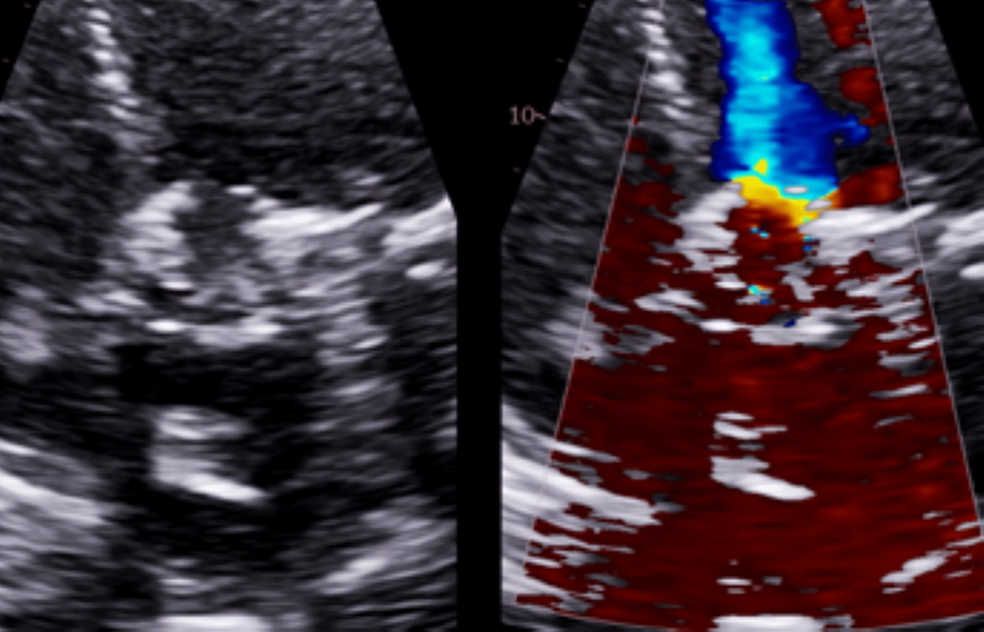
Eight Weeks Post TAVR. Thrombosed Valve while on Apixaban Ultrasound five-chamber long-axis view with color doppler showing flow acceleration across the bioprosthetic aortic valve. TAVR: transcatheter aortic valve replacement
